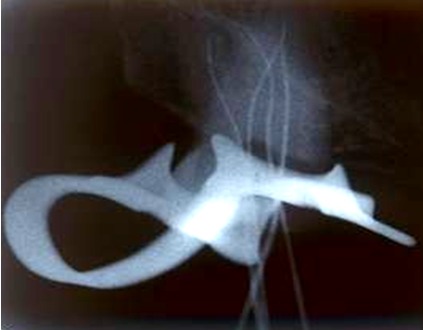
Working Length determination radiograph with four K-files.
